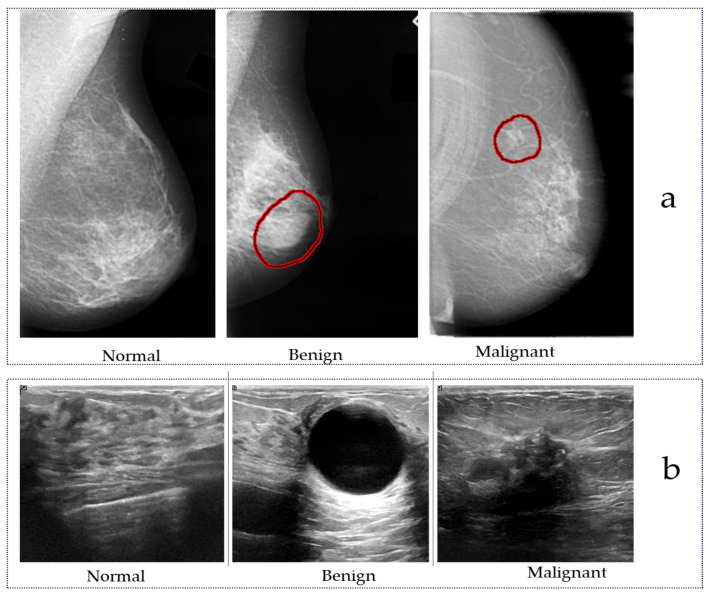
(a) A sample from the DDSM dataset [26], and (b) a sample of breast ultrasound images from the dataset [34].
